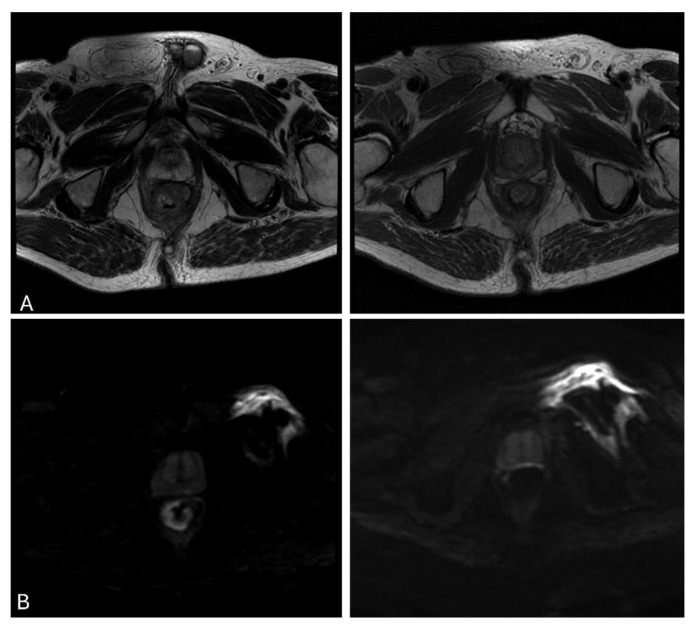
A 75-year-old male patient with low-rectal cancer, stage IIIc treated with neoadjuvant chemoradiotherapy (CTRT) and classified as a responder (mrTRG = 1). (A) Oblique axial T2-w image and (B) DWI (b-value = 1000) from the baseline examination before (right) and after neoadjuvant therapy (left).
